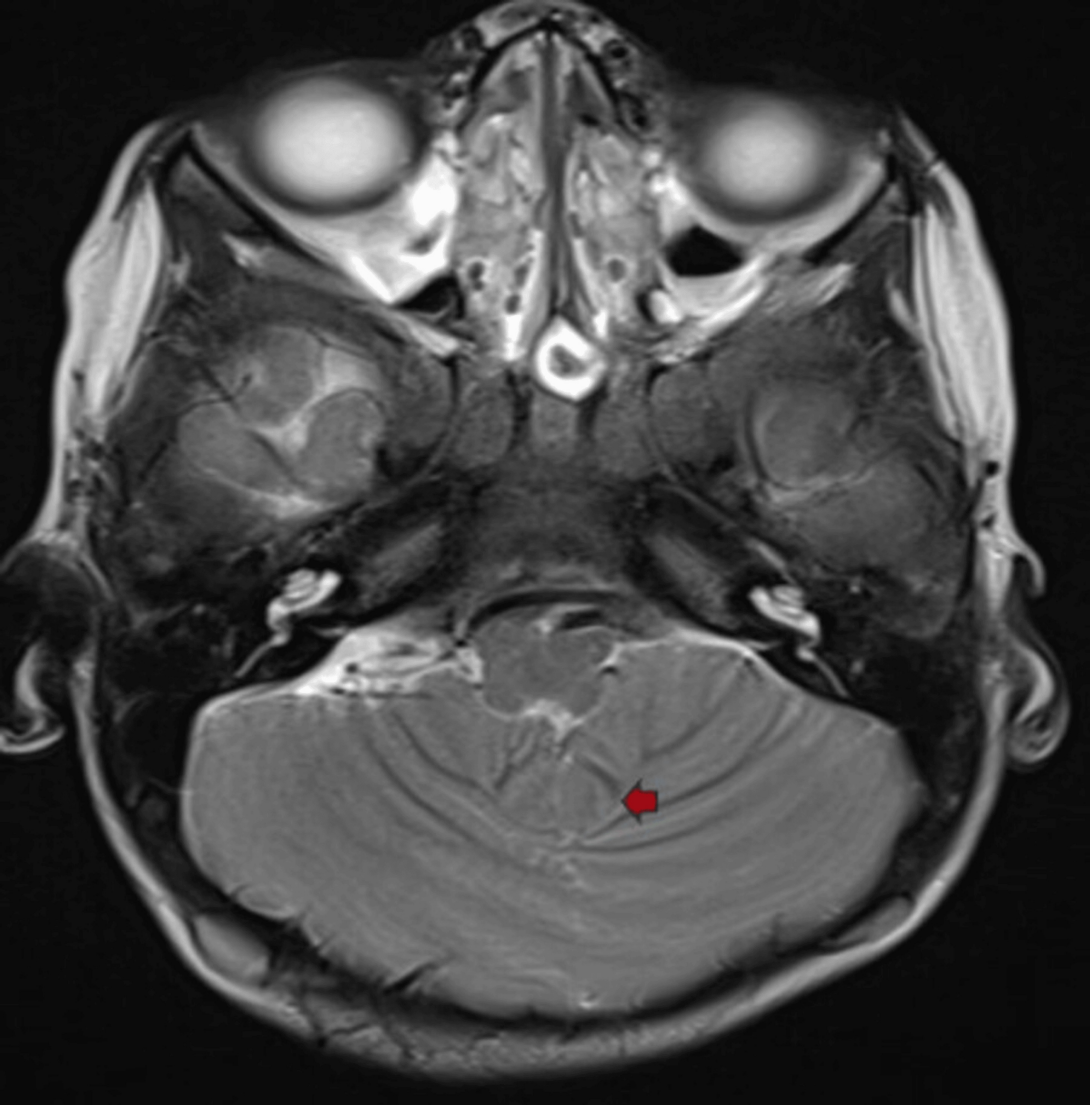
MRI axial T2WI findings The image shows a single-lobe cerebellum with vermian hypoplasia (red arrow) MRI: magnetic resonance imaging; T2WI: T2-weighted imaging
